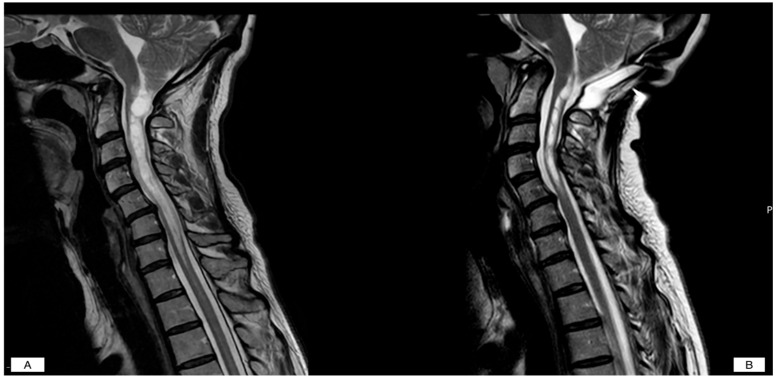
Mild clinical CM-I symptomatology with symptomatic syringomyelia. (A): Pre- and post-operative MRI scans demonstrating a significant reduction of the syrinx. (B): Post-operative MRI was obtained after 6 months.
